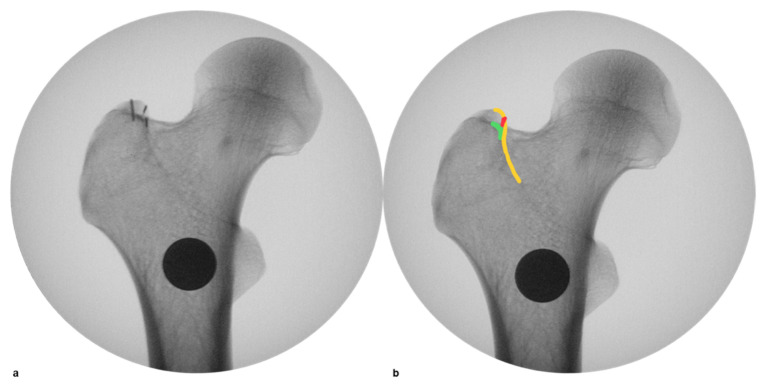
The best available anterior posterior hip image was selected based on the profile of the greater trochanter, specifically radiographic overlap of the intertrochanteric ridge and lateral wall of the piriformis fossa. (a): The antero- and postero-superior borders of the greater trochanter overlap in the Cortical Overlap View, this coincides with an overlapping of the easily recognizable intertrochanteric crest and density line of the piriform fossa. (b): Yellow marks the intertrochanteric ridge. Green marks the density line of the piriform fossa. Red marks the posterior-superior border of the greater trochanter [36].
